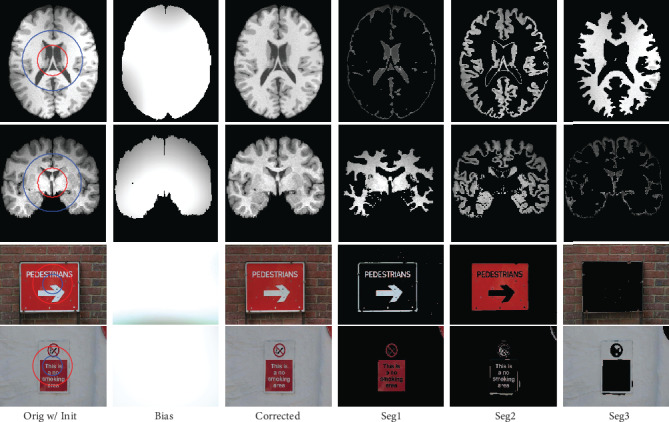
Results of GINC_1_^3^ and GINC_3_^3^ in segmeting brain MR images and natural images from MSRCORID database, respectively.
